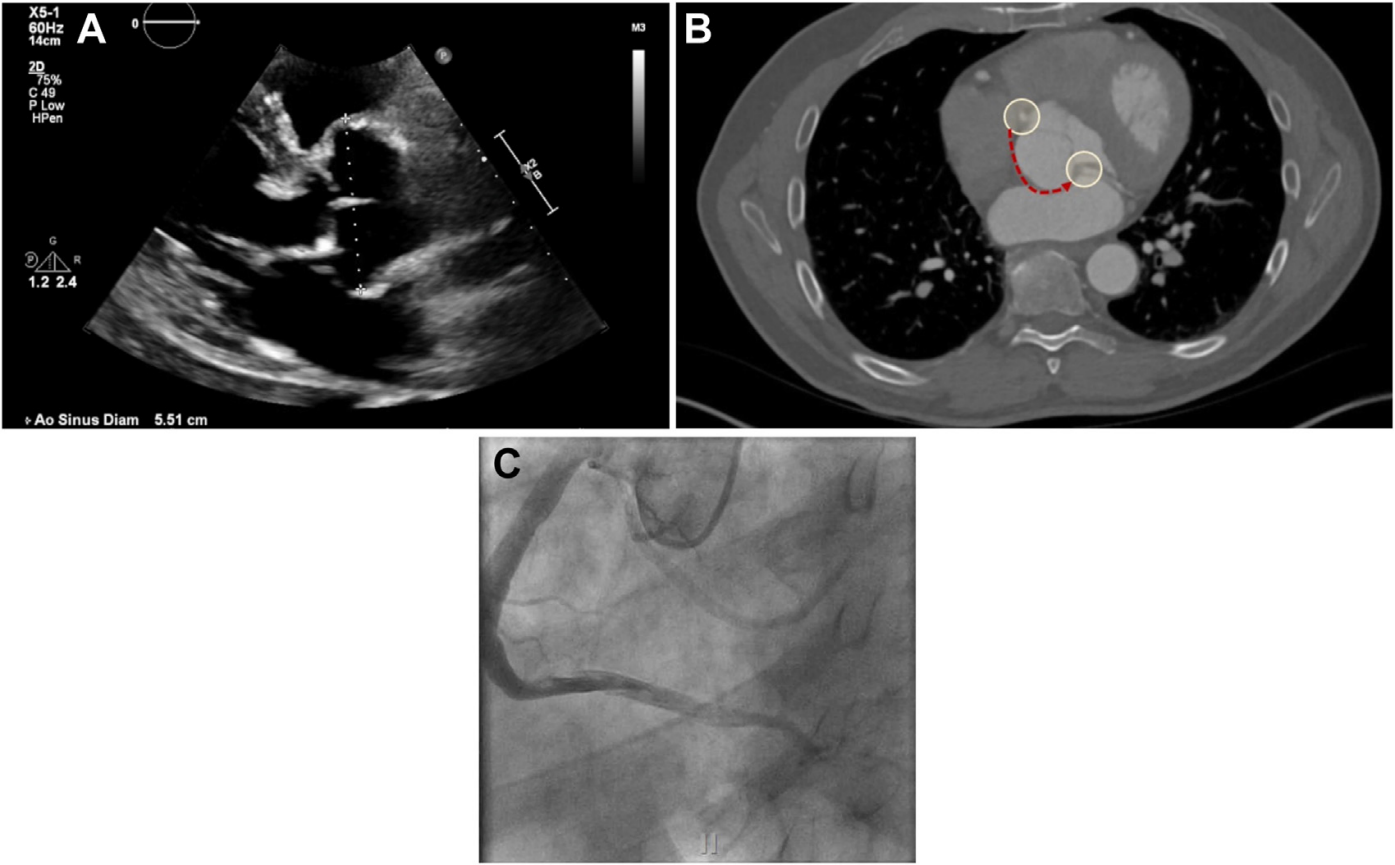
(A) Preoperative transthoracic echocardiogram. (B) Preoperative computed tomography angiography with anomalous left circumflex course highlighted. (C) Preoperative left-sided heart catheterization with opacification of the right coronary artery and the anomalous left circumflex.
